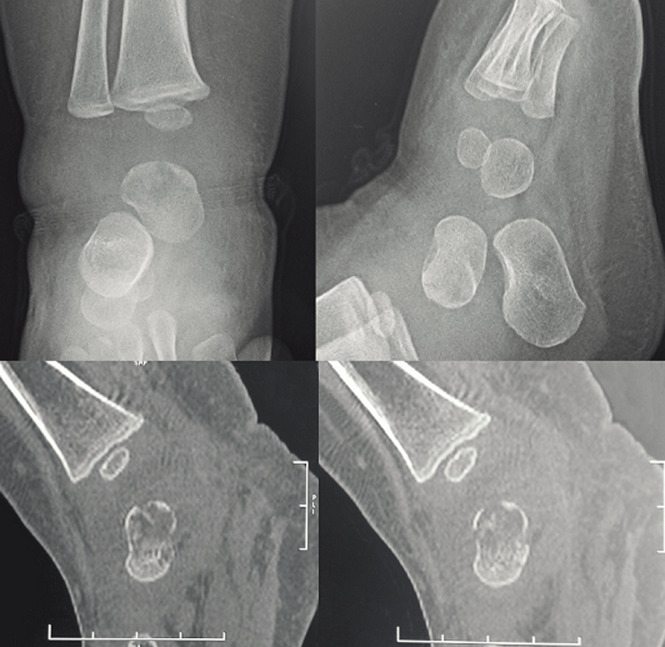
ankle imaging: X-ray of the ankle showing a lytic lesion of the talus; CT scan showing a talus fracture
